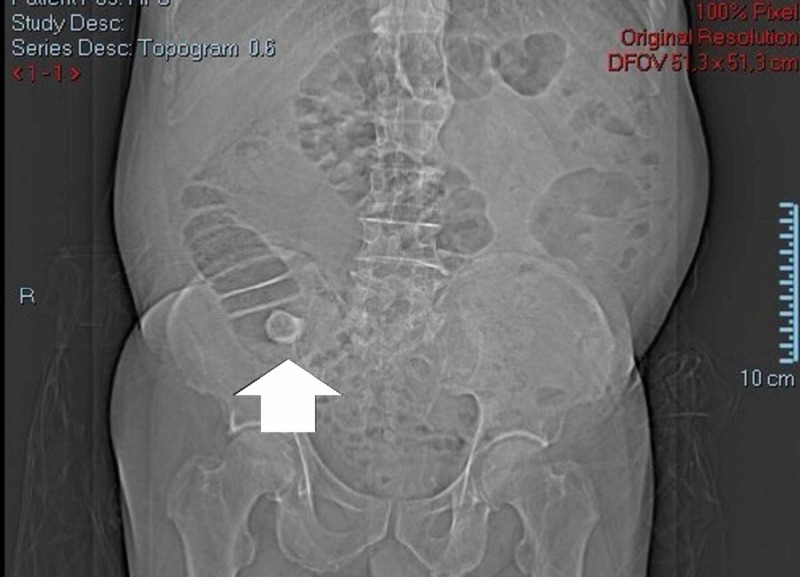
CT scan view. One of Rigler´s triad signs - presence of ectopic gallstone.
